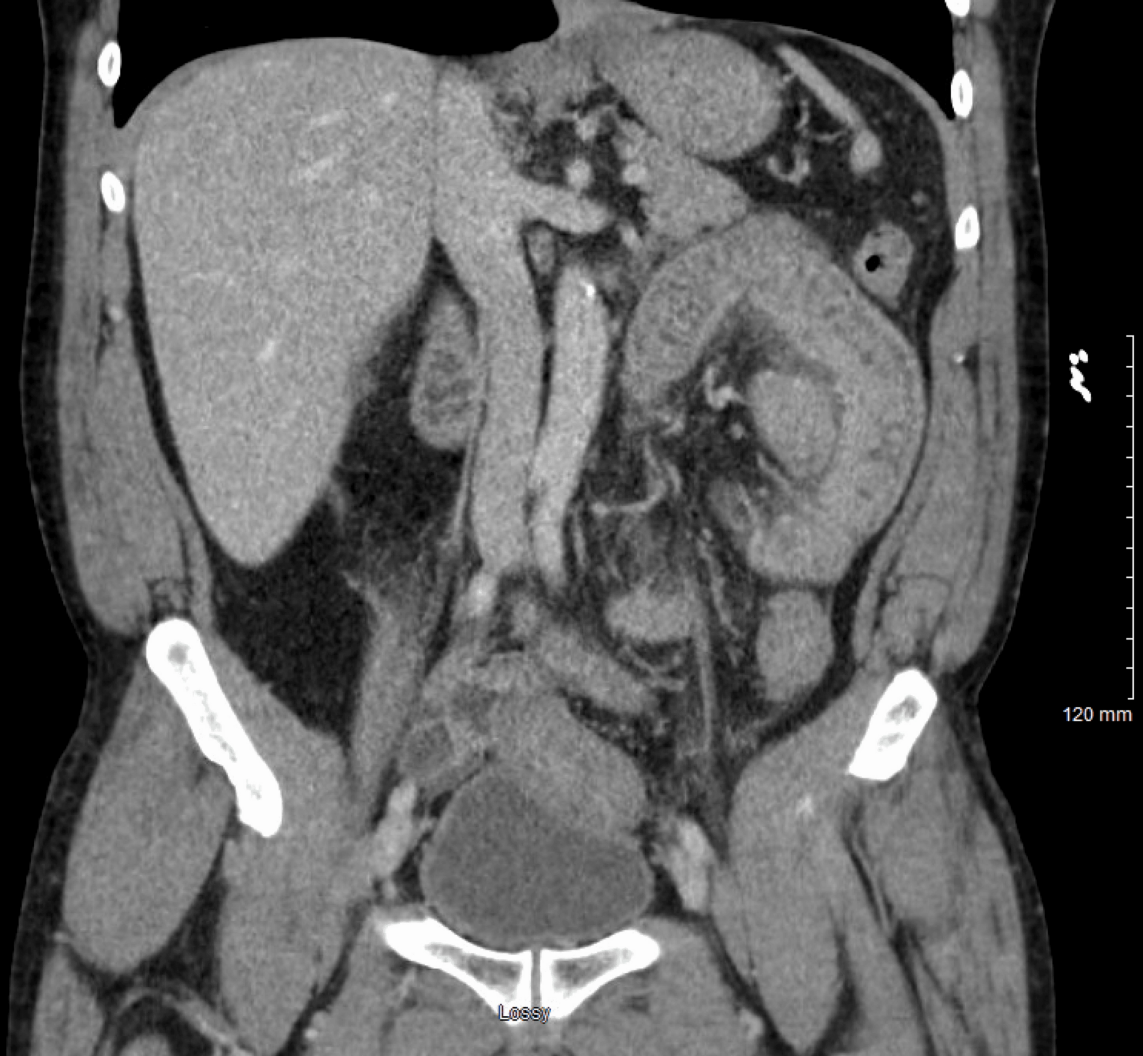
CT abdomen coronal view Demonstrated prominent thickening of the jejunum in the left upper quadrant with mucosal edema and surrounding inflammatory changes, as well as thickening of the sigmoid colon. These findings, in conjunction with adjacent free fluid (Figure 2) and the absence of obstruction, ischemia, or perforation, are characteristic of intestinal AE and help distinguish it from infectious enteritis, mesenteric ischemia, and acute diverticulitis.
